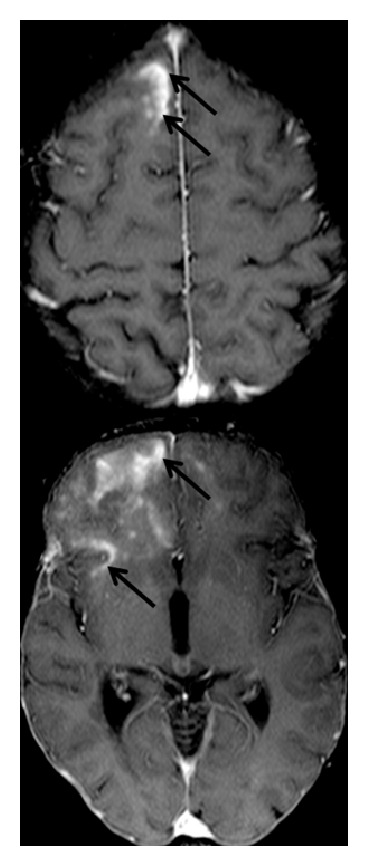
Signs of IRIS at the time of PML diagnosis. 1.5 T T1w Gadolinium enhanced MR images are presented. Extensive Gadolinium enhancement suggestive of IRIS (black arrows) was observed at the edge of confluent PML lesions.
